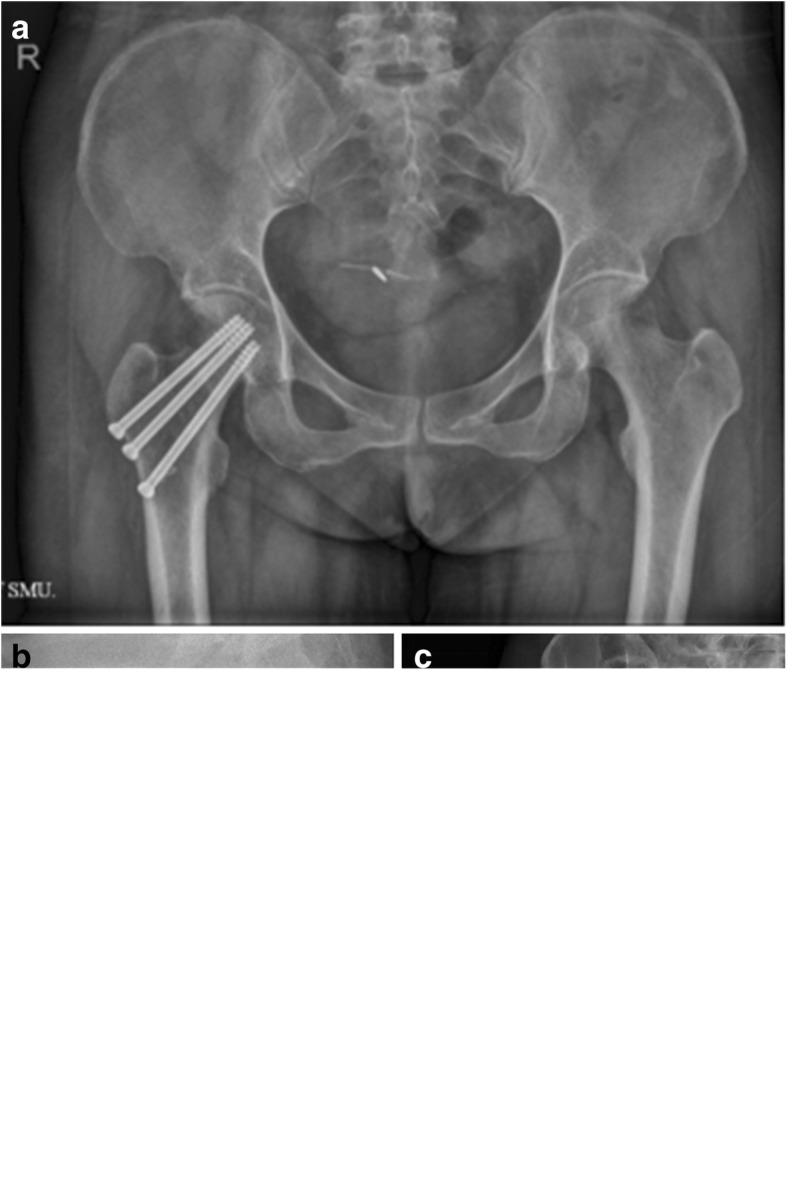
Anteroposterior view of pelvic (a) and lateral radiograph of the femoral neck (b) after closed reduction and internal fixation with three cannulated screws. Anteroposterior view of the hip after internal fixation was removed (c)
